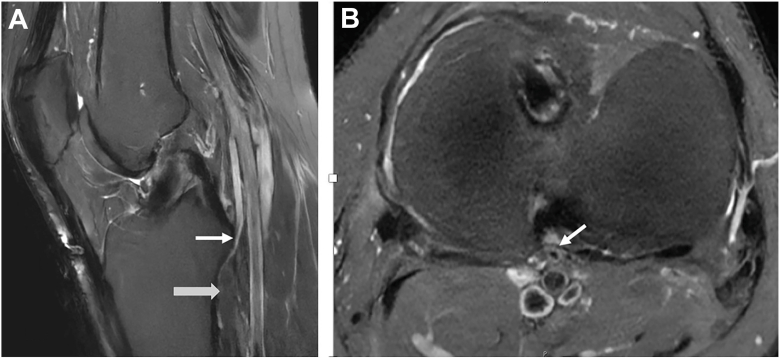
MRI example of Kim et al. 1989 type II-A2. (a) Sagittal T2-weighted image showing anterior tibial artery (white arrow) coursing anterior to the popliteus muscle (gray arrow). (b) Axial T2-weighted image showing the aberrant anterior tibial artery (white arrow) immediately adjacent to the distal-posterior aspect of the posterior cruciate ligament, before coursing along the posterior tibial cortex.
